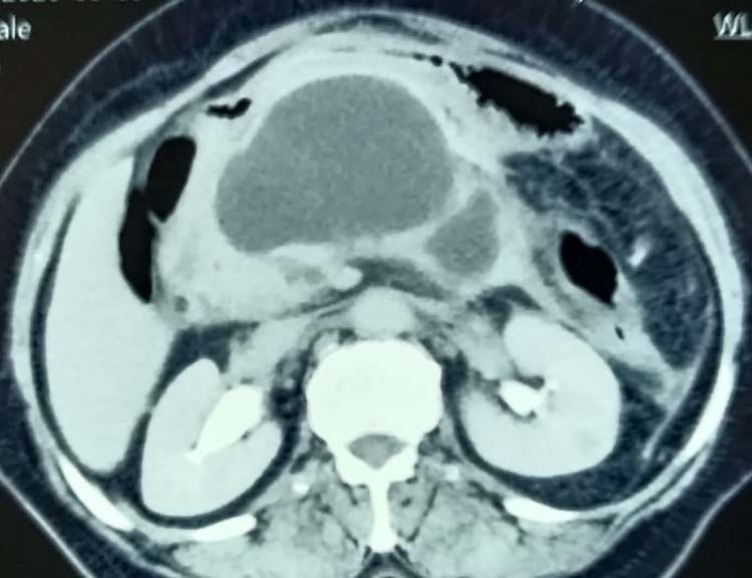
Contrast CT abdomen showing walled-off pancreatic necrosis in the pancreatic head, close to the distal bile duct.
